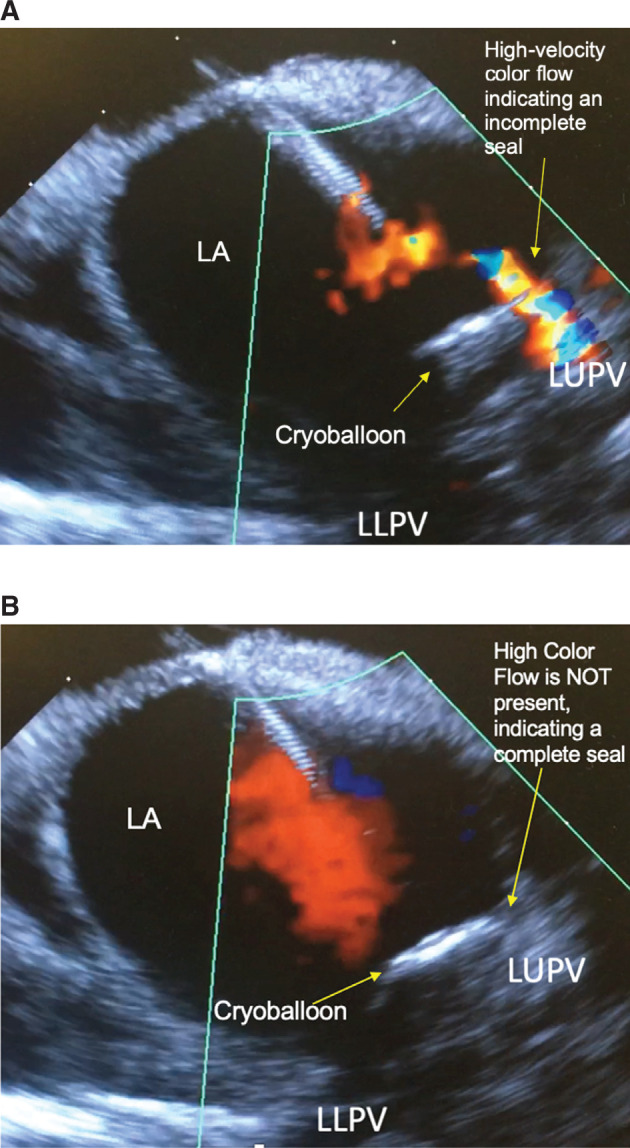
A: ICE image of the LA. The CB partially occluded the flow around the periphery of the vein. Note the presence of Doppler color flow around the right side of the CB. B: ICE image of the LA. CB fully occluded flow around the periphery of the vein. Note the absence of Doppler color flow around the right side of the balloon. CB: cryoballoon; ICE: intracardiac echo; LA: left atrium; LLPV: left lower pulmonary vein; LUPV: left upper pulmonary vein.
